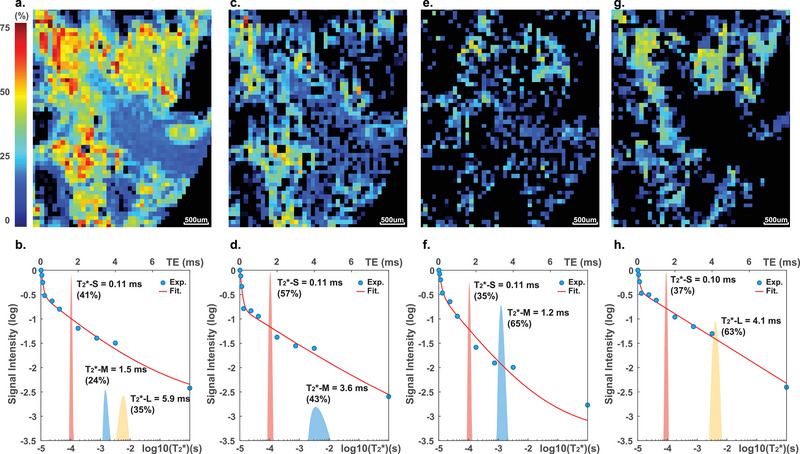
Ultrahigh‐resolution MRI of human pericortical tissue with osteoarthritis (OA‐1). a) 2D ^1^H intensity color map from a UTE image at a resolution of 62.5 µm (field of view: 8×8×20 mm^3^, matrix size: 128×128×128). b) T_2_* distribution of the whole cross‐section. c) Color map of the T_2_*‐S fraction and d) T_2_* distribution of the same region. The T_2_*‐S region represents the collagen matrix compartment. e) Color map of the T_2_*‐M fraction and f) T_2_* distribution of the same region. The T_2_*‐M region represents the free pore water compartment. g) Color map of the T_2_*‐L fraction and h) T_2_* distribution of the same region. The T_2_*‐L region represents the lipid compartment.
